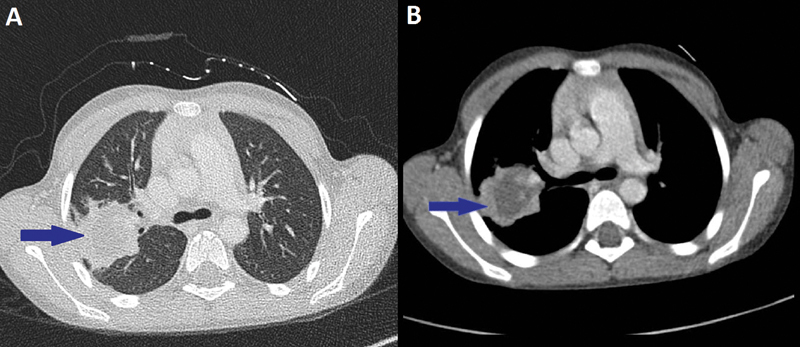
Chest computed tomographic scan showing a right cystic pulmonary parenchymal mass (arrow) in both lung window (
